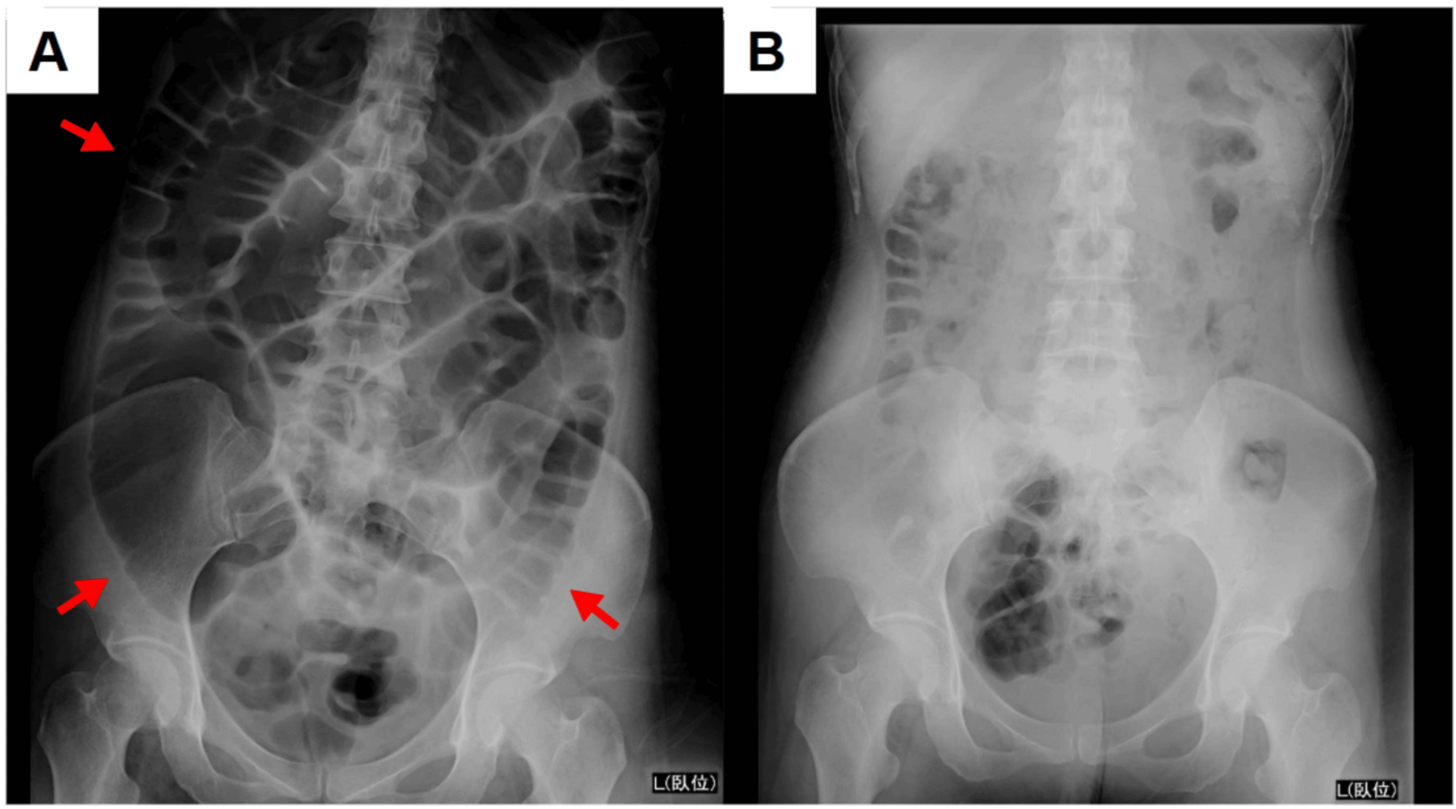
Abdominal X-rays showing paralytic ileus Radiography performed on day 4 (A) shows diffuse intestinal dilation without evidence of mechanical obstruction, which improved with conservative treatment by day 14 (B).
